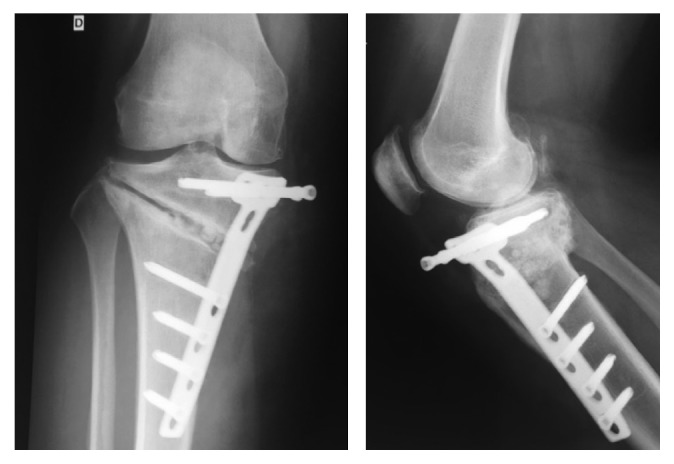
Early June 2010, 1 year after HTO, postoperative X-rays showing an important pullout of the proximal screws and slight translation of the proximal fragment of the HTO.
